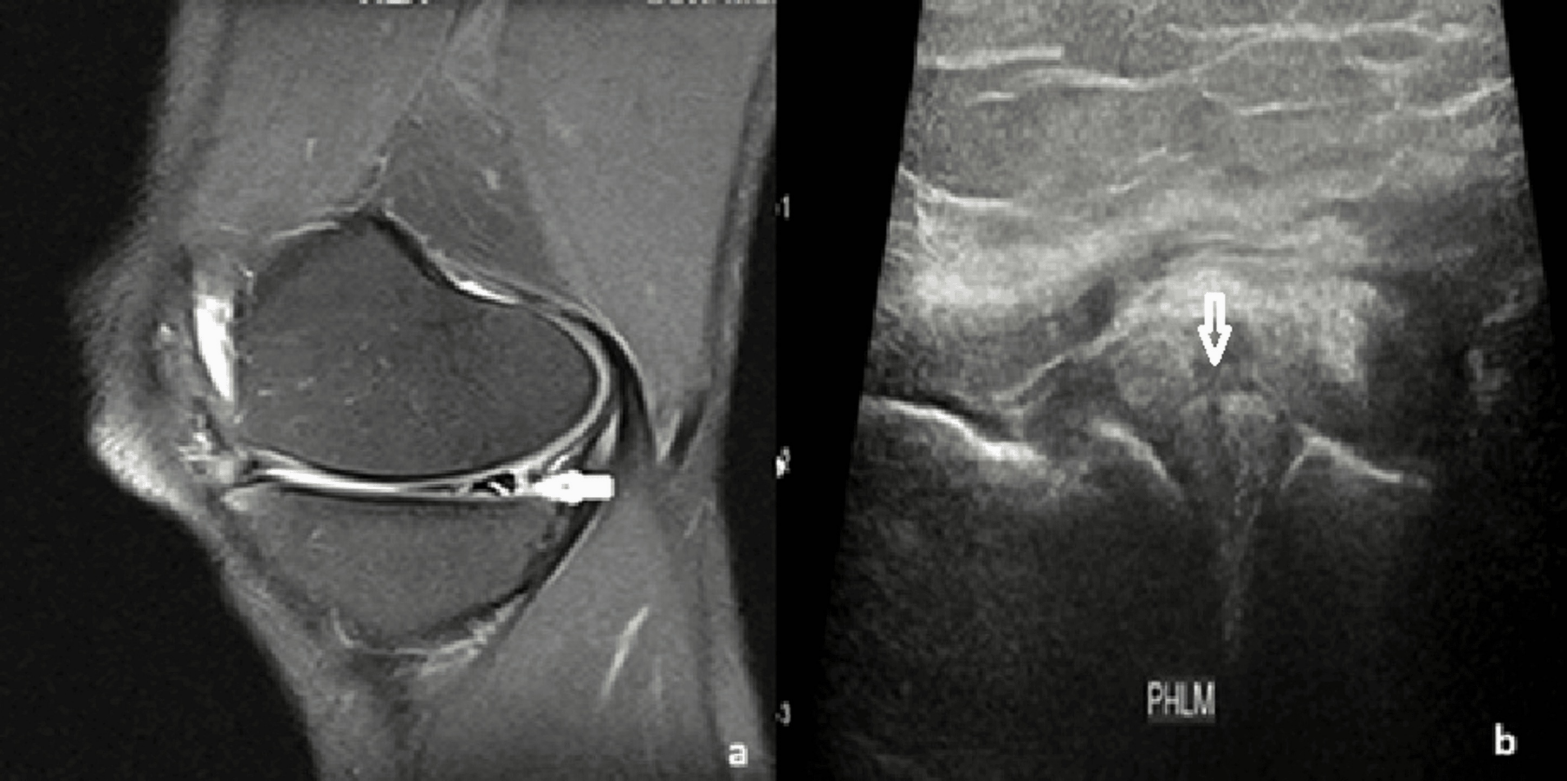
A 21-year-old male patient with a vertical tear of the lateral meniscus. (a) Sagittal PDFS MRI image demonstrating a vertical tear extending to the inferior articular surface of the lateral meniscus. (b) Corresponding USG image demonstrating a linear hypoechogenicity within the normally echogenic lateral meniscus, consistent with a tear. PDFS: proton density fat suppression; USG: ultrasonography; MRI: magnetic resonance imaging
